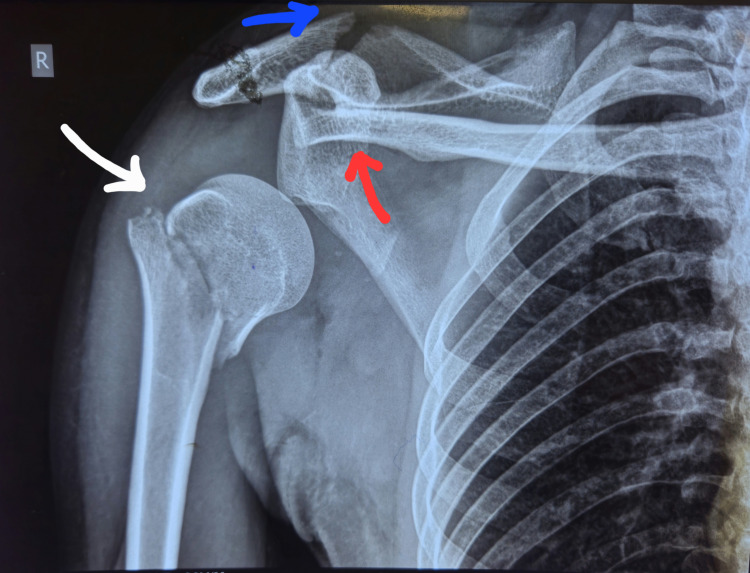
Preoperative radiograph of the affected shoulder demonstrating a displaced Neer two-part proximal humerus fracture with anterior-inferior humeral head dislocation (white arrow), ipsilateral Rockwood type VIb (subcoracoid) acromioclavicular joint dislocation (red arrow), and minimally displaced scapular spine fracture (blue arrow).
